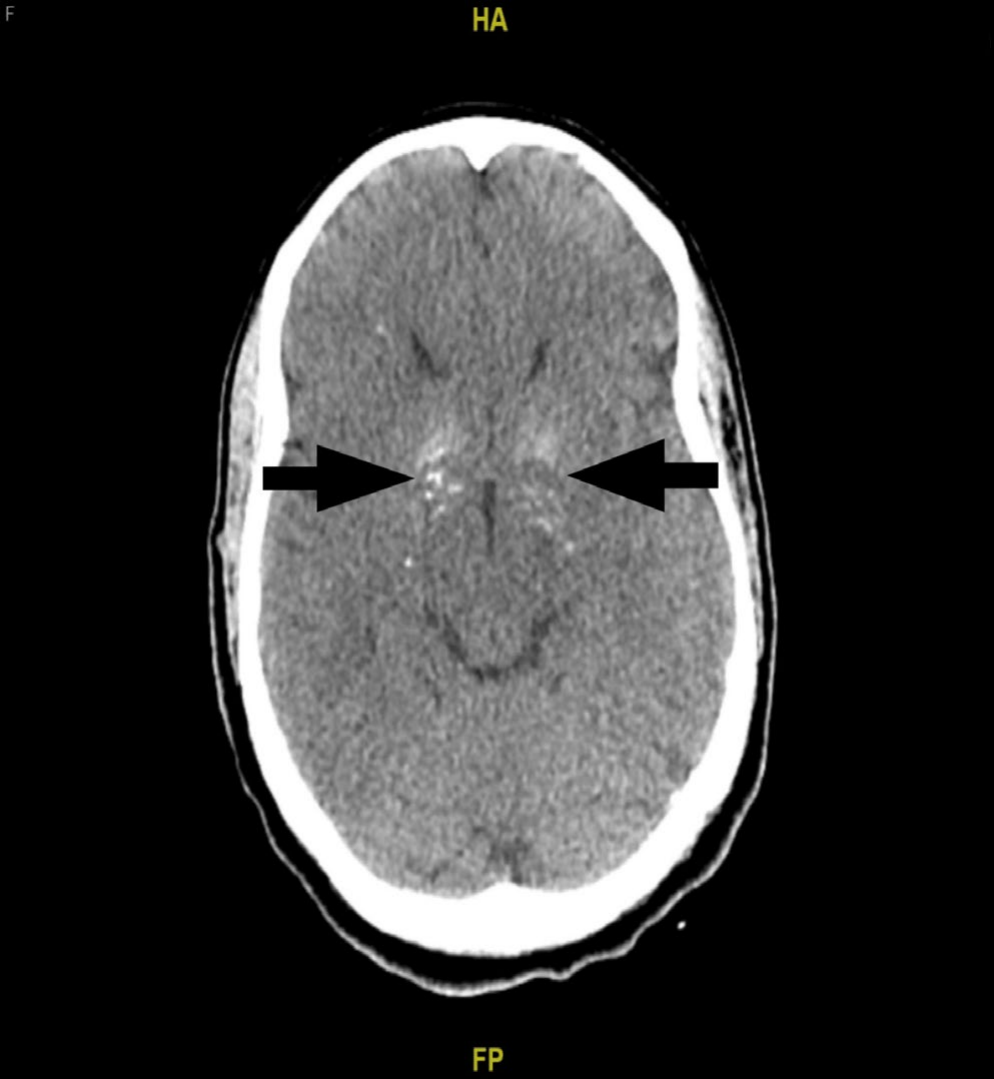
Brain CT scan showing bilateral basal ganglia calcification presenting as hyperdense areas in the basal ganglia region.
